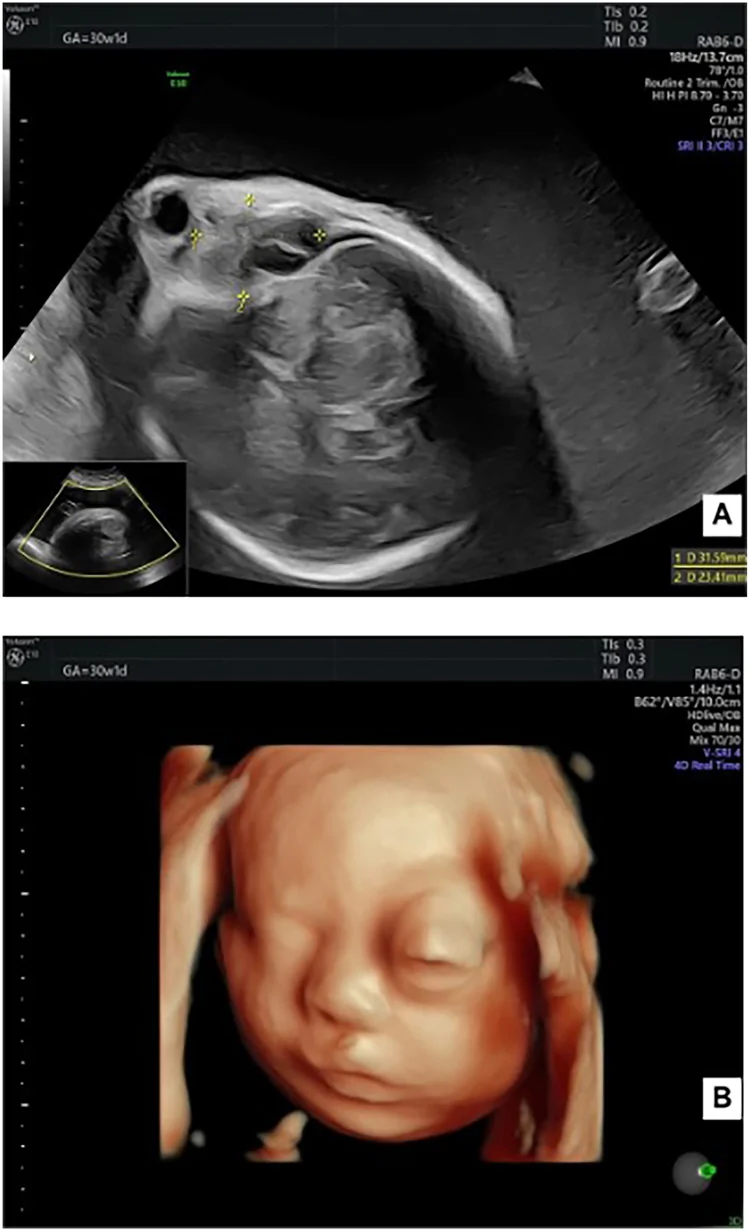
Fetal ultrasound findings at 30 weeks of gestation. (A) A solid-cystic mass extending toward the left retroorbital area, with heterogeneous content. (B) Three-dimensional image of the anterior compression of the facial region caused by the same lesion, as seen on 4D ultrasound.
